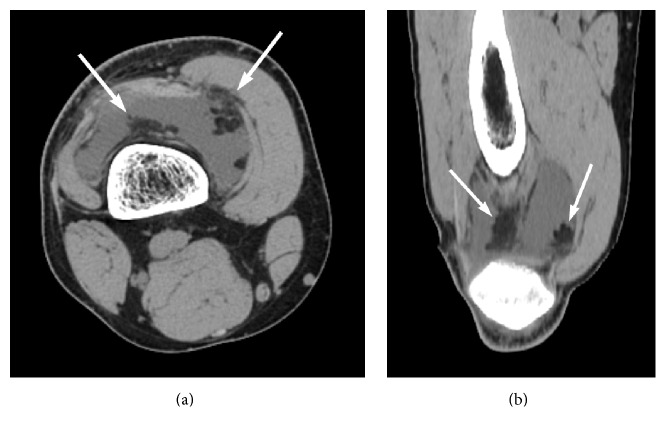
CT images on axial (a) and reconstructed coronal (b) plane in soft tissue window reveal a low-density intra-articular mass similar to fat with multiple villi projecting inwards (arrows), which are surrounded by joint fluid with higher density than fat but lower than water. Distention of the joint capsule is evident.
